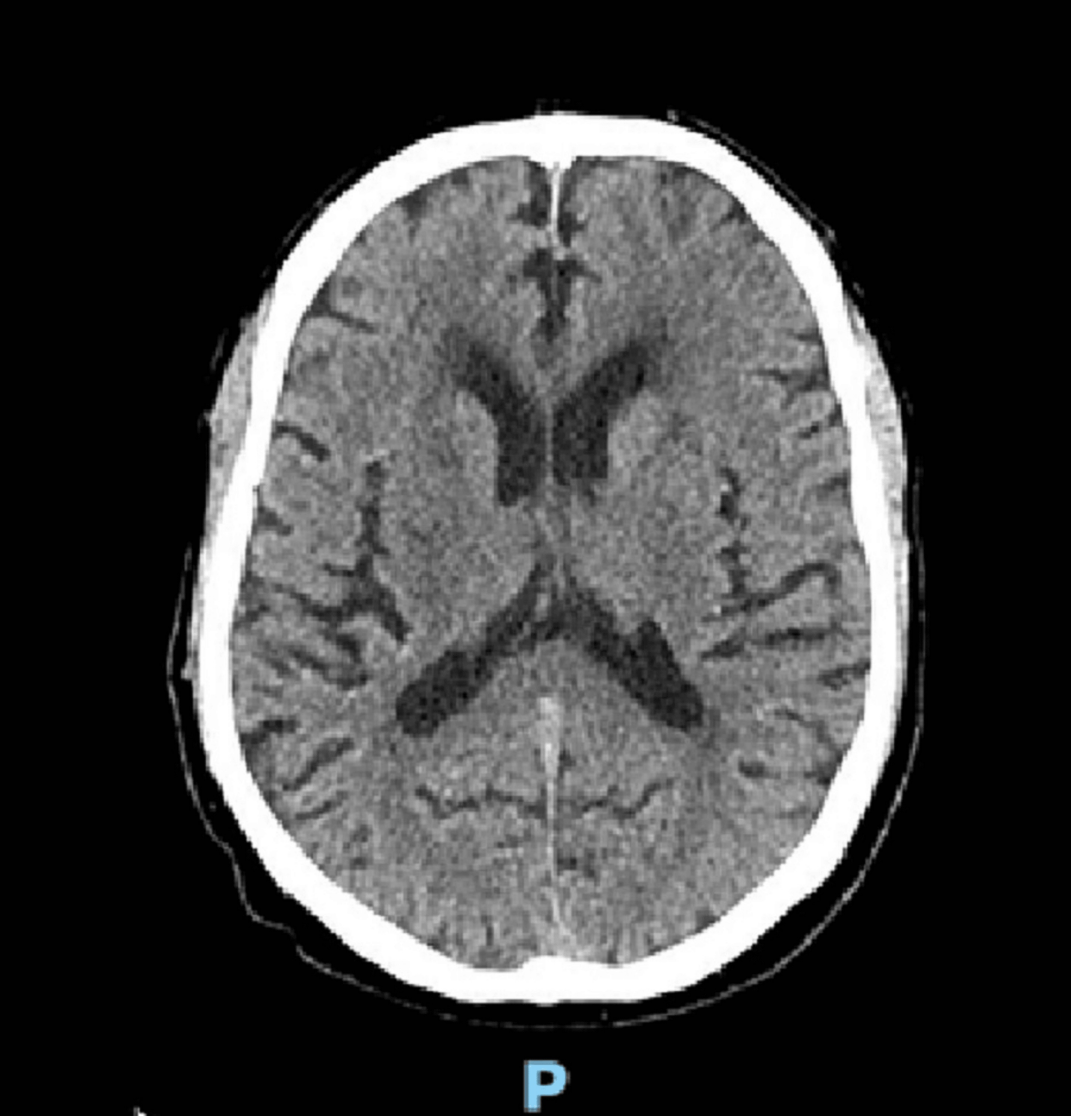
CT of the brain: no acute lesions.
